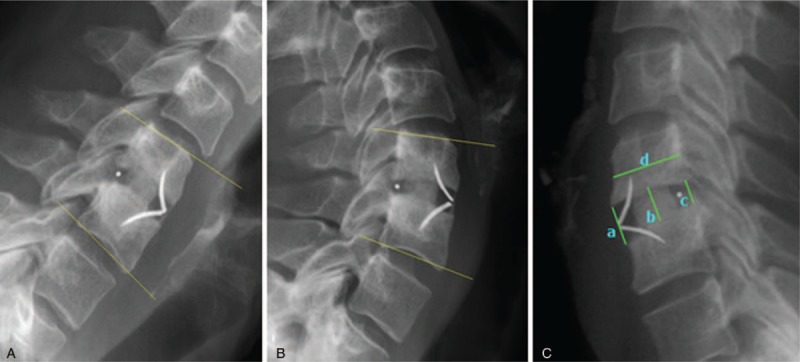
(A) Functional spinal unit alignment in flexion. (B) Functional spinal unit alignment in extension. (C) Radiographic measurements of disc height: (a) Anterior disc height, (b) Middle disc height, (c) Posterior disc height, (d) Sagittal diameter of the overlying vertebral body. Disc height index = [(a + b + c)/3]/d.
